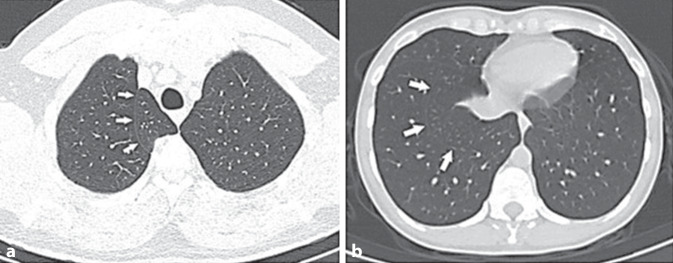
a Accessory azygos fissure on the right (white arrows indicate the fissure; transverse CT image). b Accessory fissure between the medial basal segment and anterior basal segment of the lower lobe on the right (white arrows indicate the fissure; transverse CT image)
